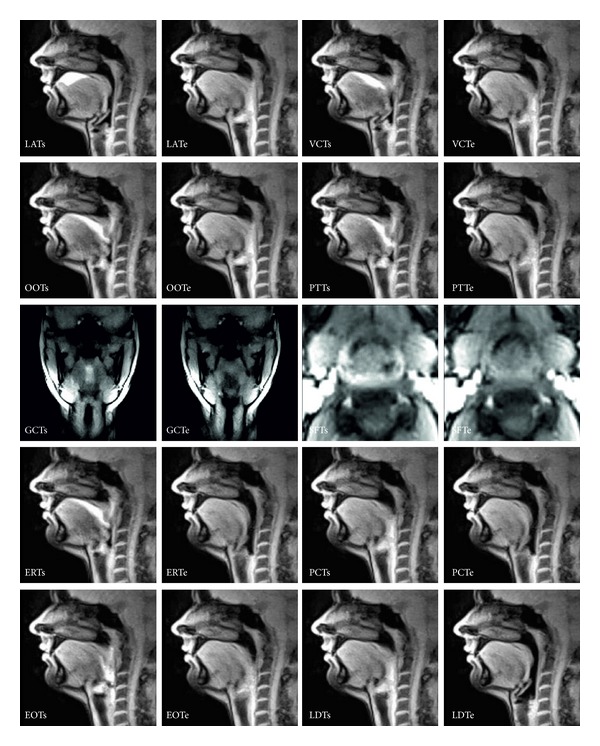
Physiological events of normal swallowing as seen by real-time MRI (27-year-old female). LAT: laryngeal ascent, VCT: velo-pharyngeal closure, OOT: oro-velar opening (start time defined as reference), PTT: pharyngeal transit, GCT: glottal closure, SFT: vallecular and piriform sinus filling, ERT: epiglottic retroflexion, PCT: pharyngeal constriction, EOT: esophageal opening, LDT: laryngeal descent (“s” and “e” refer to respective start and end times). The images are selected from respective movies (see Supplementary Movie 1 in the Supplementary Material available online at http://dx.doi.org/10.1155/2014/493174) at a resolution of 41.2 ms (24.3 frames per second) and sorted according to their temporal onset from top left to bottom right. For further details see text.
